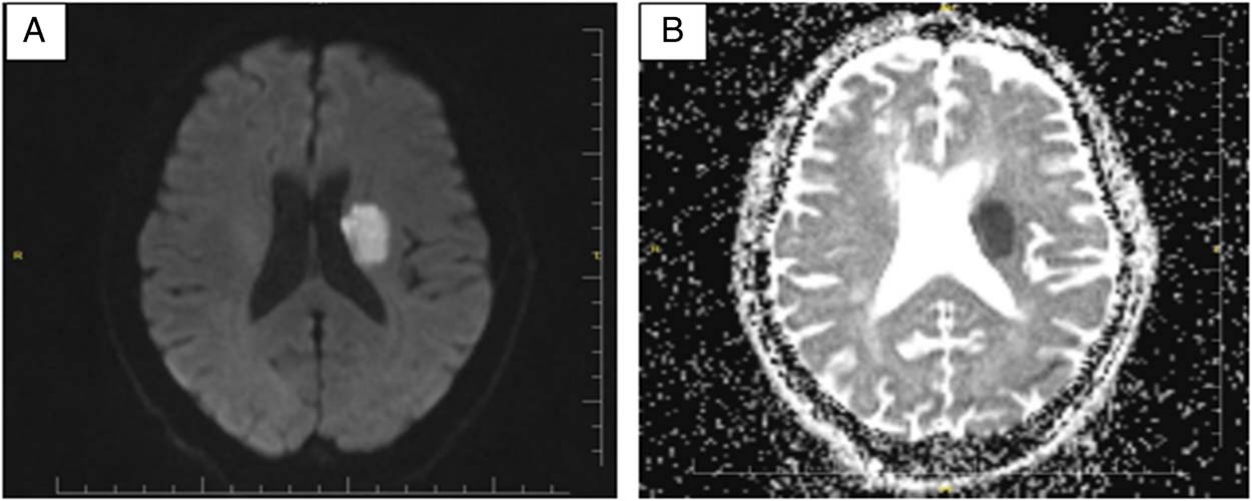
(A, B) Diffusion brain MRI showing diffusion restriction (hyperintensity in diffusion-weighted imaging and hypointensity in apparent diffusion coefficient) in the left basal ganglion consistent with an acute infarct.
